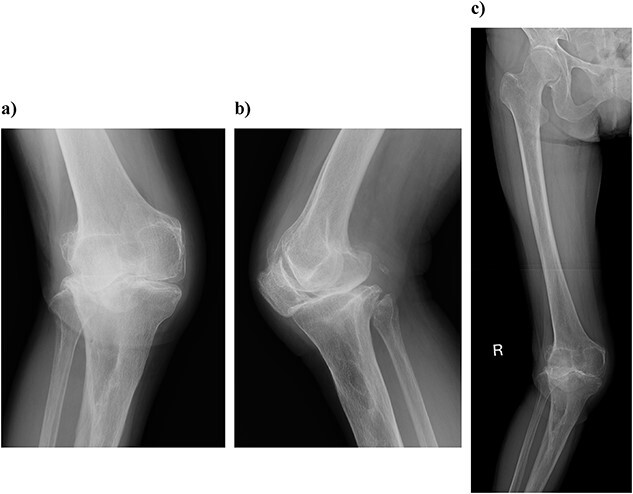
Plain radiography at the patient’s first visit: (a) standing front, (b) lateral side and (c) thigh, standing, front, full length. Valgus knee osteoarthritis and well-defined osteoporosis near the central part of the proximal tibia can be seen, and the rough surface of the tibia has been worn away.
